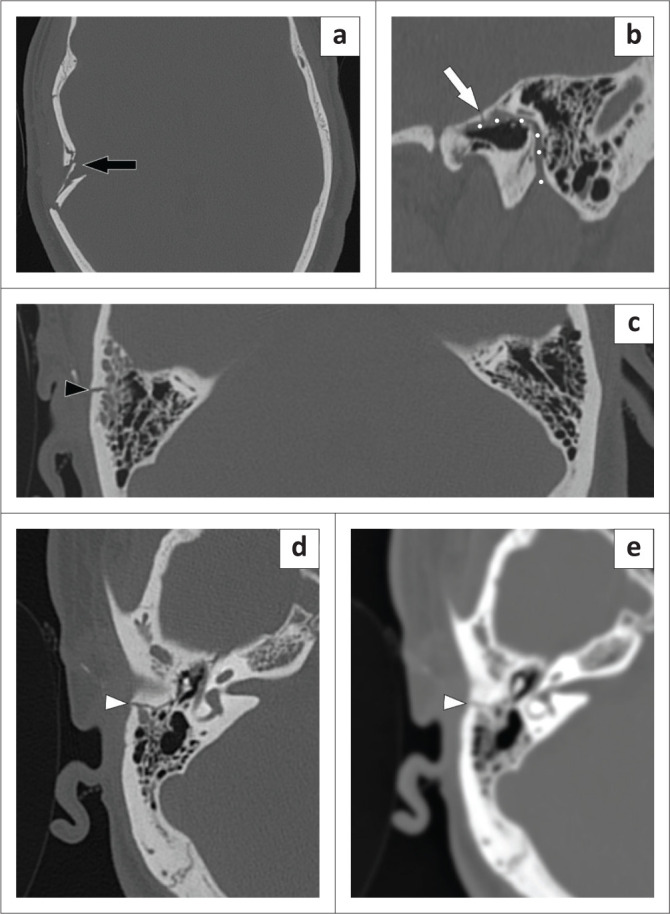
Axial CT of the head (a) demonstrating the depressed right squamous temporal bone fracture (black arrow) detected on initial interpretation. An oblique reconstruction of the right temporal bone (b) shows that the fracture (white arrow) extends into the facial nerve canal (highlighted here by the white dots). Specifically, it involves the tympanic segment of the canal. The fracture line extending into the mastoid part of the temporal bone is pointed out by the black arrowhead in the axial image (c) – note the fluid in the mastoid air cells and compare it to the well-aerated left mastoid air cell; a secondary sign of temporal bone fracture. The fracture line (white arrowhead) is much more conspicuous on the axial cut of the bone reconstruction algorithm (d) than the soft tissue algorithm (e), despite both being set to a standard ‘bone window’.
